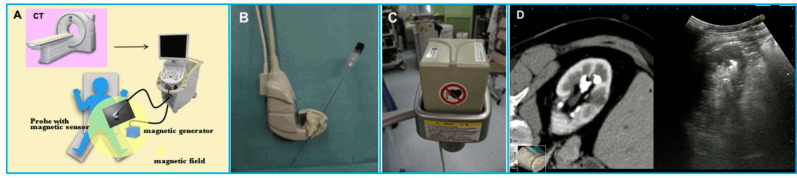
A navigation system for percutaneous renal puncture. (A) The main components of the system; (B) The magnetic sensor attached to an US probe; (C) The magnetic field generator; (D) US image and CT volume data displaying side by side on the same monitor. Reprinted from [175] with permission.
